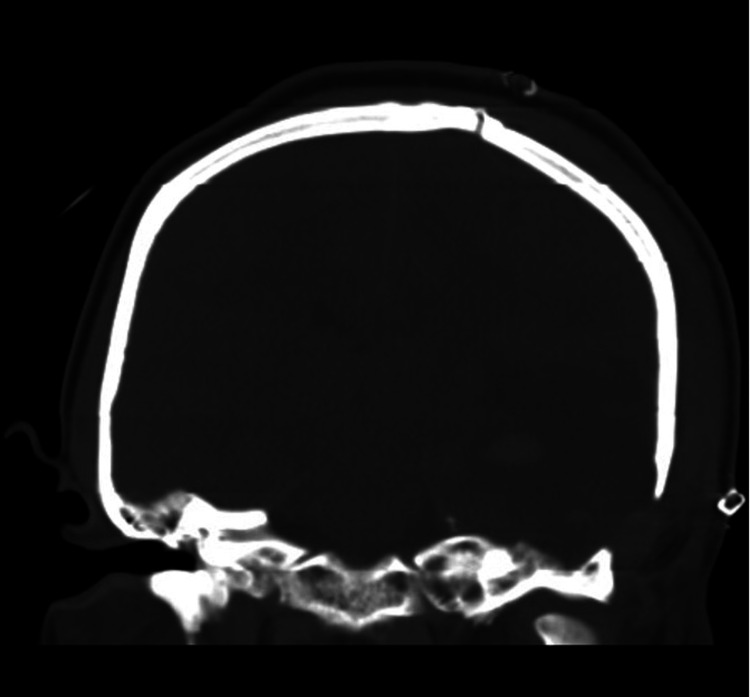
Coronal CT bone window showing the expansion of the bone flap with the use of the hinge. Image courtesy Dr. Salazar Jones.
